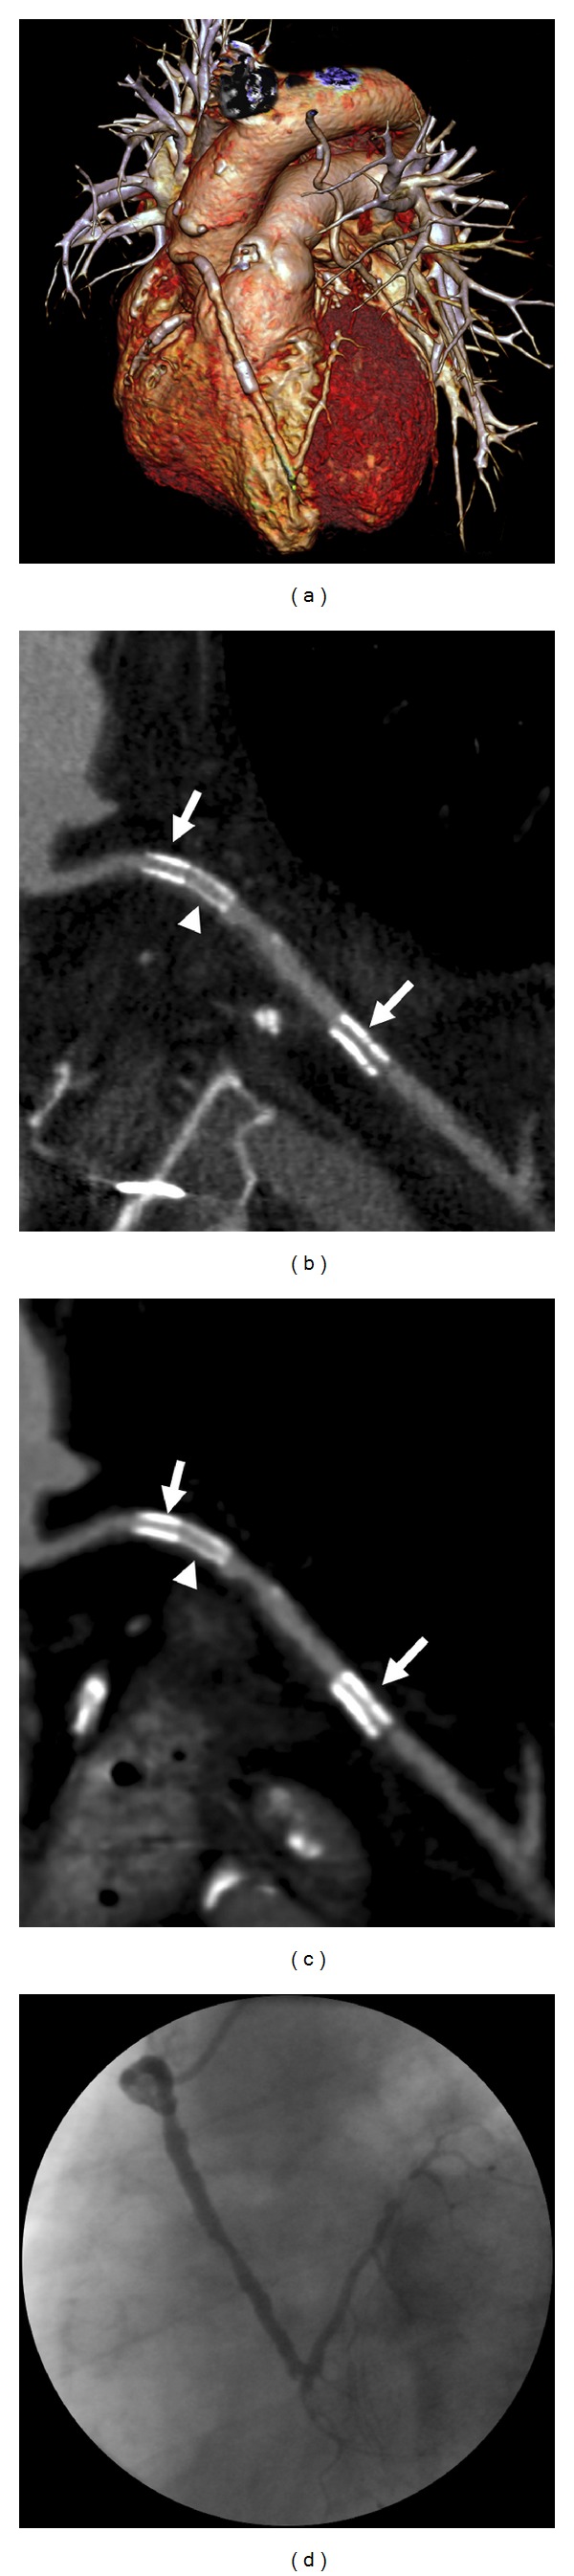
62-year-old male patient with a history of myocardial infarction and surgical revascularization therapy. 14 years after surgery, he developed CABG stenoses and subsequent stenting. CT was performed for ruling ISR. The 3D-volume rendered CT image shows the course of the stented vein graft to the LAD. A left internal mammary artery graft to a marginal branch is also depicted (a). Multiplanar curved reformats reconstructed with a dedicated sharp convolution kernel (b) and a smooth standard convolution kernel for cardiac CT angiography (c) show three CABG stents with a nominal diameter of 3 mm each. There are two TAXUS stents with 132 *μ*m strut thickness (arrows) and a Xience V stent with 81 *μ*m strut thickness (arrowhead). There is notably more blooming with the thicker struts and the stent lumen is better visible with the dedicated convolution kernel. ISR was ruled out by CT. This finding was confirmed by coronary angiography (d).
